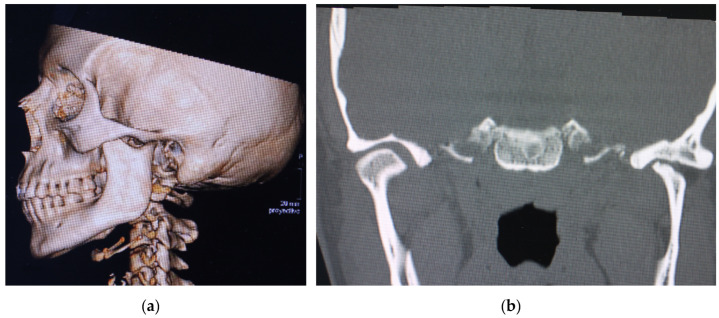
Preoperative computed tomography. (a) Lateral view; (b) flattening and degeneration of the left mandibular condyle.
